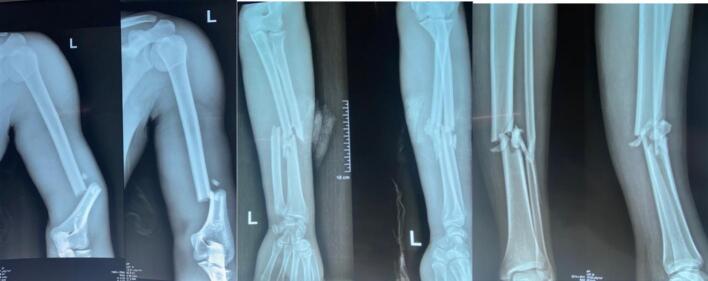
Plain X-rays of the arm, forearm and ipsilateral leg.
